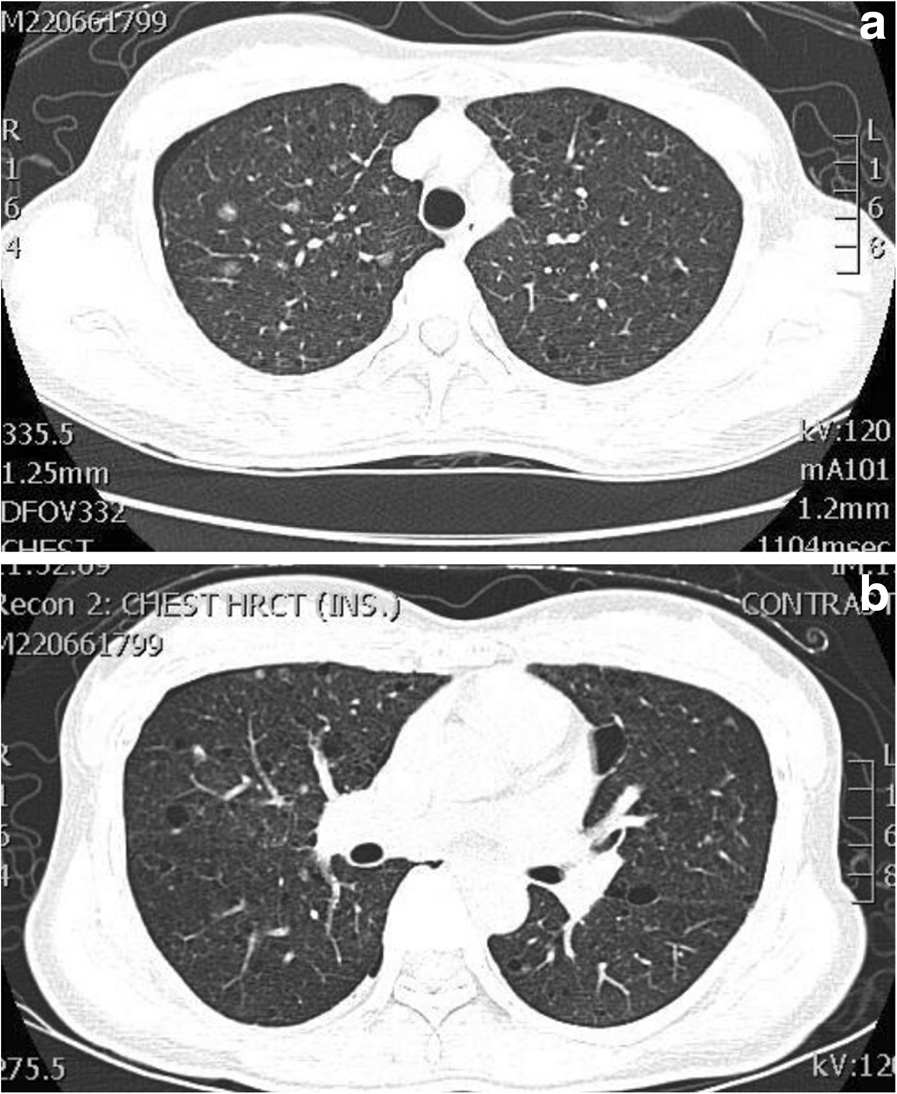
a and b Chest HRCT. HRCT showed bilateral numerous cystic lesions with right small pulmonary nodules.
